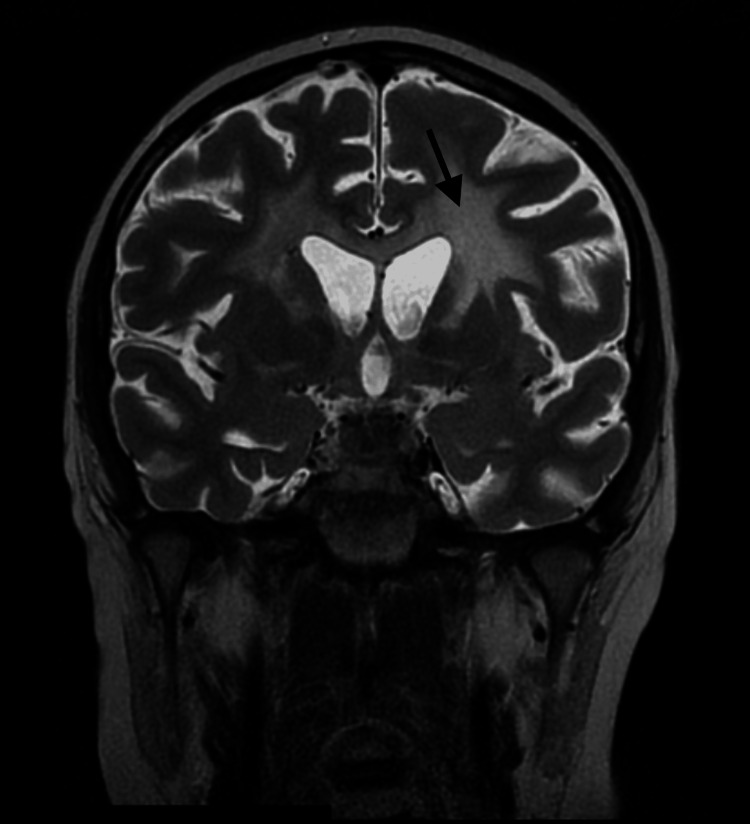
Brain MRI (T2-weighted coronal image) showing subcortical white matter hyperintensity. MRI: Magnetic Resonance Imaging
